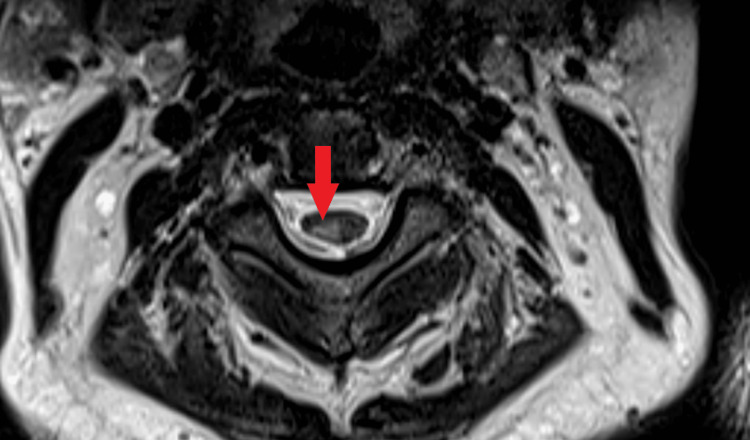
T2-weighted axial image showing hyperintense signal in the cervical cord on the right side.
